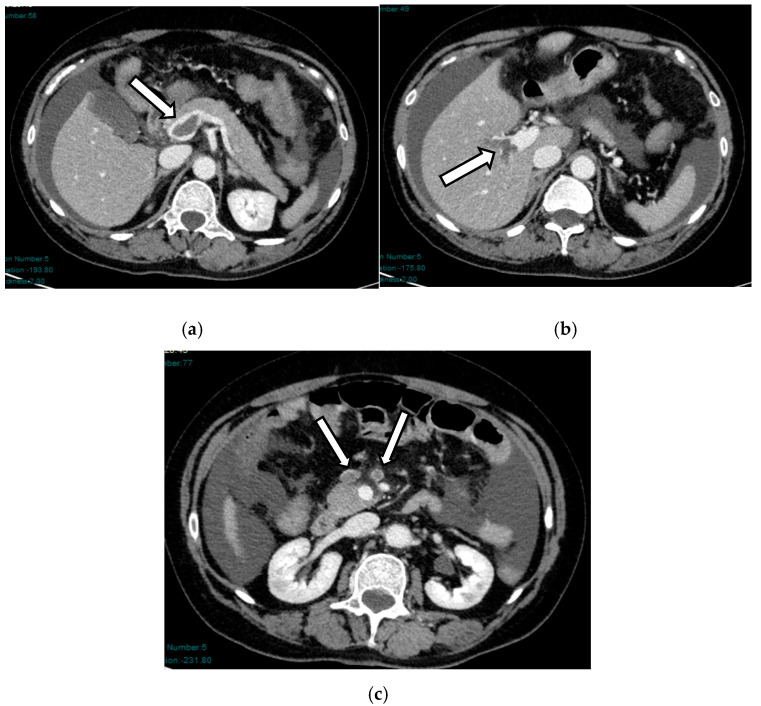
Thrombotic AMVI in a 64-year-old woman: axial post-contrast CT scans show large intraluminal filling defects (pointed out by arrows) in the portal vein (markedly dilated) (a), in the intrahepatic portal branches (b), and in the SMV and inferior mesenteric vein (IMV) (c).
